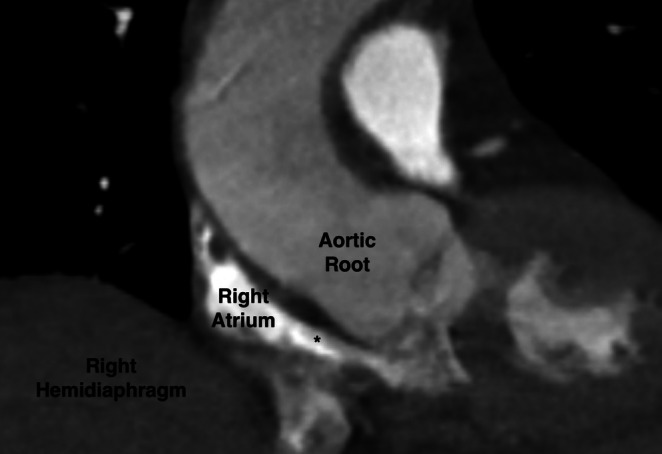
CT scan demonstrating the compression of the right atrium against the aortic root due to elevation of the right hemidiaphragm.
